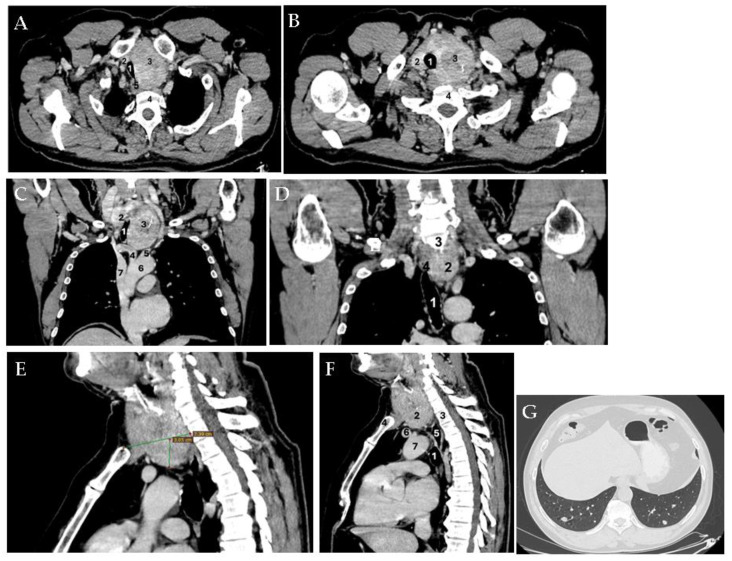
Since there was no hyperthyroidism, intravenous contrast-enhanced computed tomography (CT) was performed, which confirmed the voluminous goiter with posterior extension towards the vertebral bodies. ((A). Axial plane: 1—compressed trachea; 2—right thyroid lobe; 3—left thyroid lobe; 4—cervical spine; 5—deviated esophagus. (B). Axial plane: 1—deviated trachea; 2—right thyroid lobe; 3—left thyroid lobe; 4—cervical spine). The left internal jugular vein and the left carotid artery were displaced to the left (without a cleavage plane from these vascular structures). ((C). Coronal plane: 1—compressed trachea; 2—right thyroid lobe; 3—left thyroid lobe; 4—brachiocephalic trunk; 5—left common carotid artery; 6—aortic arch; 7—superior cave vein. (D). Coronal plane: 1—deviated trachea; 2—thyroid mass; 3—C7 cervical vertebra; 4—deviated esophagus). The thyroid mass caudally extended on the left paratracheal side, reaching the upper mediastinum in contact with the trachea, deviating it to the right and narrowing it to a diameter of 1.53 by 2.66 cm. It also caused the left lateral deviation of the mediastinal vessels. ((E). Sagittal plane: The space occupying the mass in the left thyroid lobe and isthmus with a 3.05 cm extension (vertical line) from the superior thoracic aperture (horizontal line) into the mediastinum is shown. (F). Sagittal plane: 1—trachea; 2—left thyroid lobe; 3—T2 thoracic vertebra corresponding to the lower pole of the tumor; 4—manubrium; 5—esophagus; 6—left venous brachiocephalic trunk; 7—aortic arch). The goiter had various diameters at different sections; for instance, at the level of the cricoid cartilage, diameters were 4.84 by 5.14 cm, respectively. At the clavicular level, diameters were 7 by 7.26 cm. The isthmus protruded at the level of the jugular notch (of 3 by 4.4 cm), which is situated para-tracheal on the left and posterior to the manubrium of 4.22 by 4.82 cm (the longitudinal extension reached up to 10 cm cranio-caudally). This large substernal goiter was iodophilic and showed a heterogeneous structure both on non-contrast and contrast-enhanced CT phases, while also revealing micro-calcifications and macro-calcifications. Moreover, the patient presented multiple lung micro-nodules, which were disseminated bilaterally. ((G). Lung window; axial plane). The abdominal and cerebral CT scans were normal.
